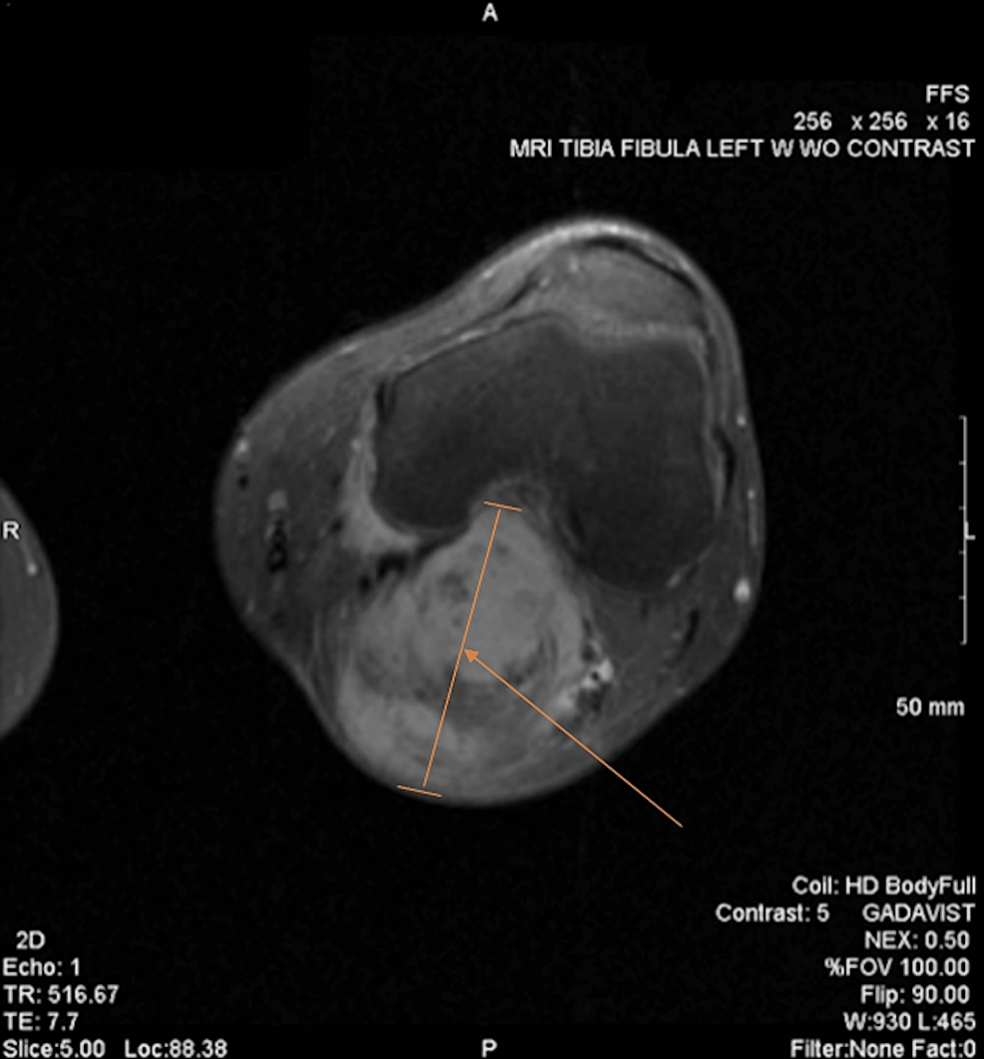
MRI (done in August 2020) demonstrating a transverse cut through the patella and distal end of the femur of the left leg. The arrow points to a line indicating the anterior-posterior length of the mass.
